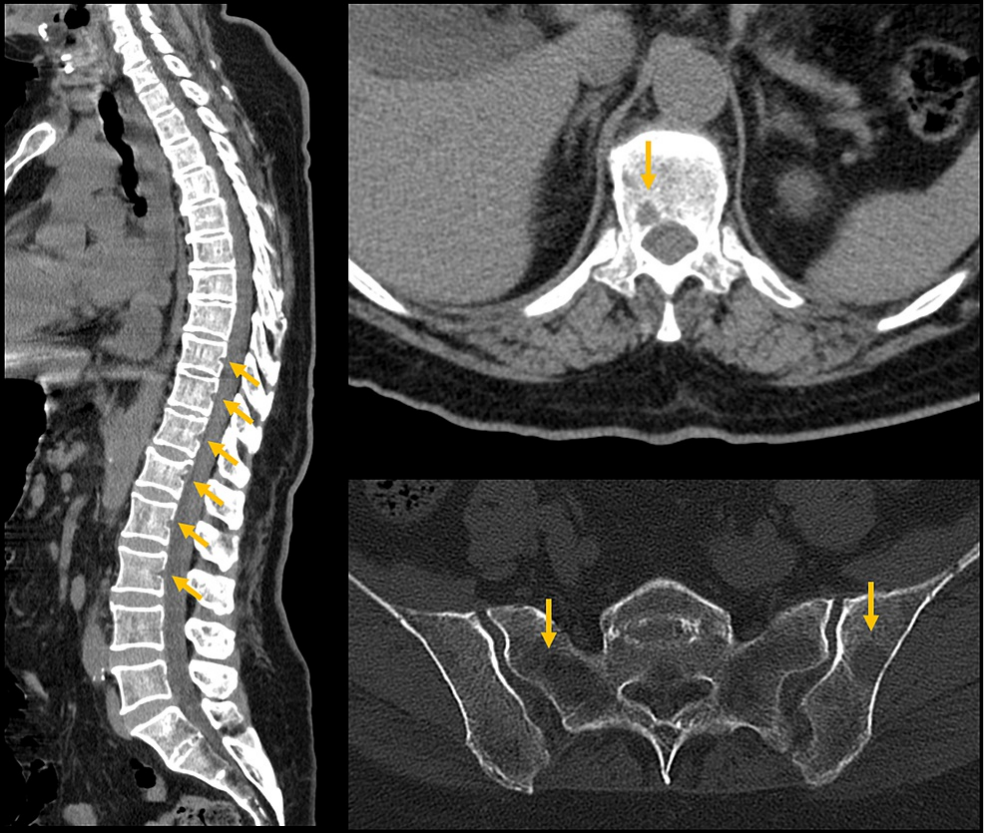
Lumbar CT showing multiple lytic lesions in vertebral bodies, sacrum, and iliac bones (yellow arrows).
